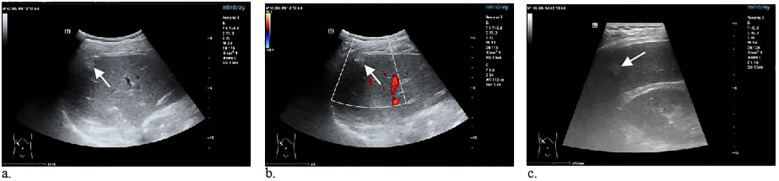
(a, b) Greyscale ultrasound (US) image shows a slightly hyperechoic nodule under the pericardium with poorly defined borders and regular morphology. Color Doppler US image shows no significant blood flow signal(arrows). (c) High-frequency US image shows a slightly hyperechoic nodule in the right posterior lobe of the liver(arrow).
